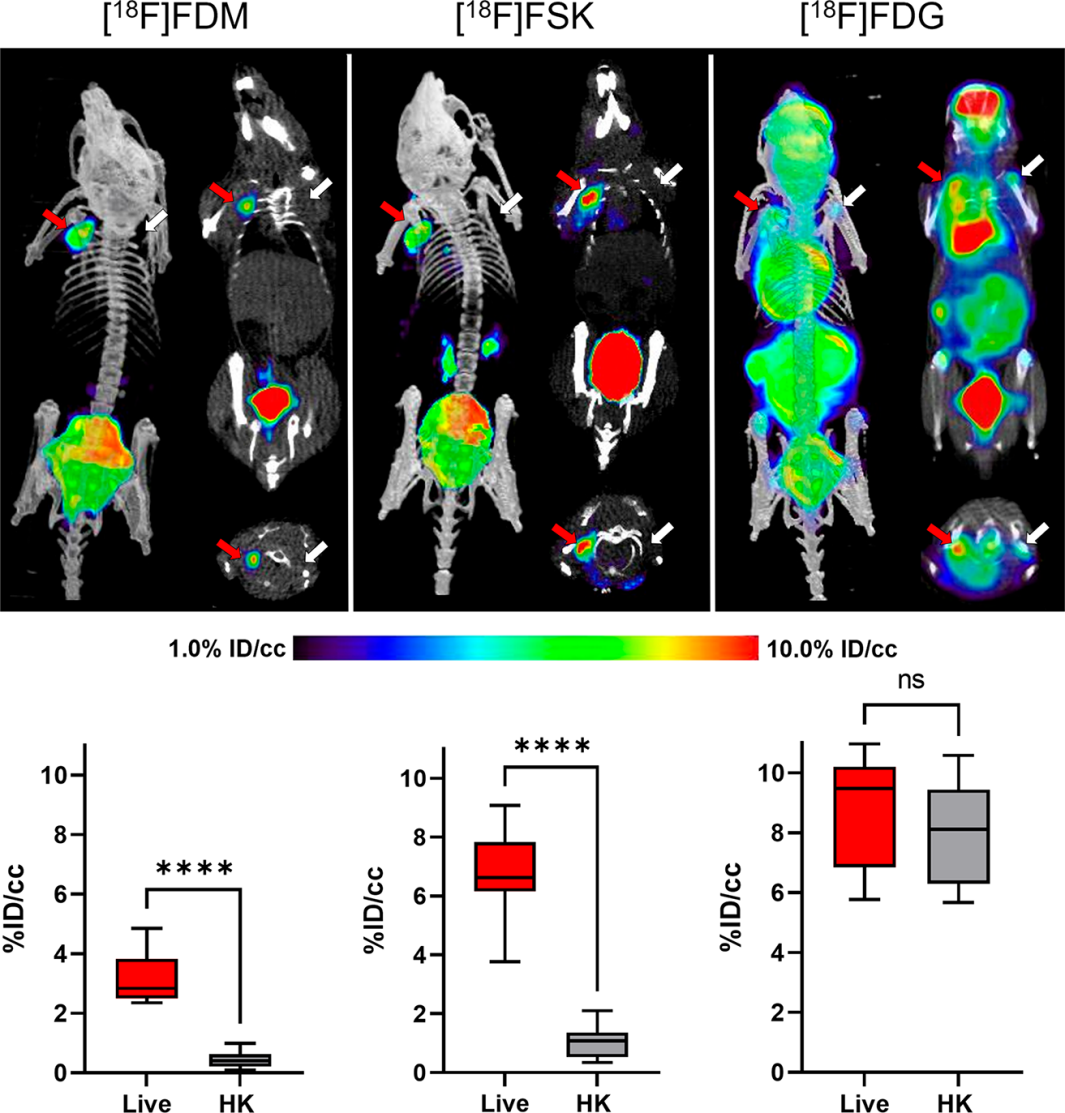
μPET-CT imaging of MRSA myositis
